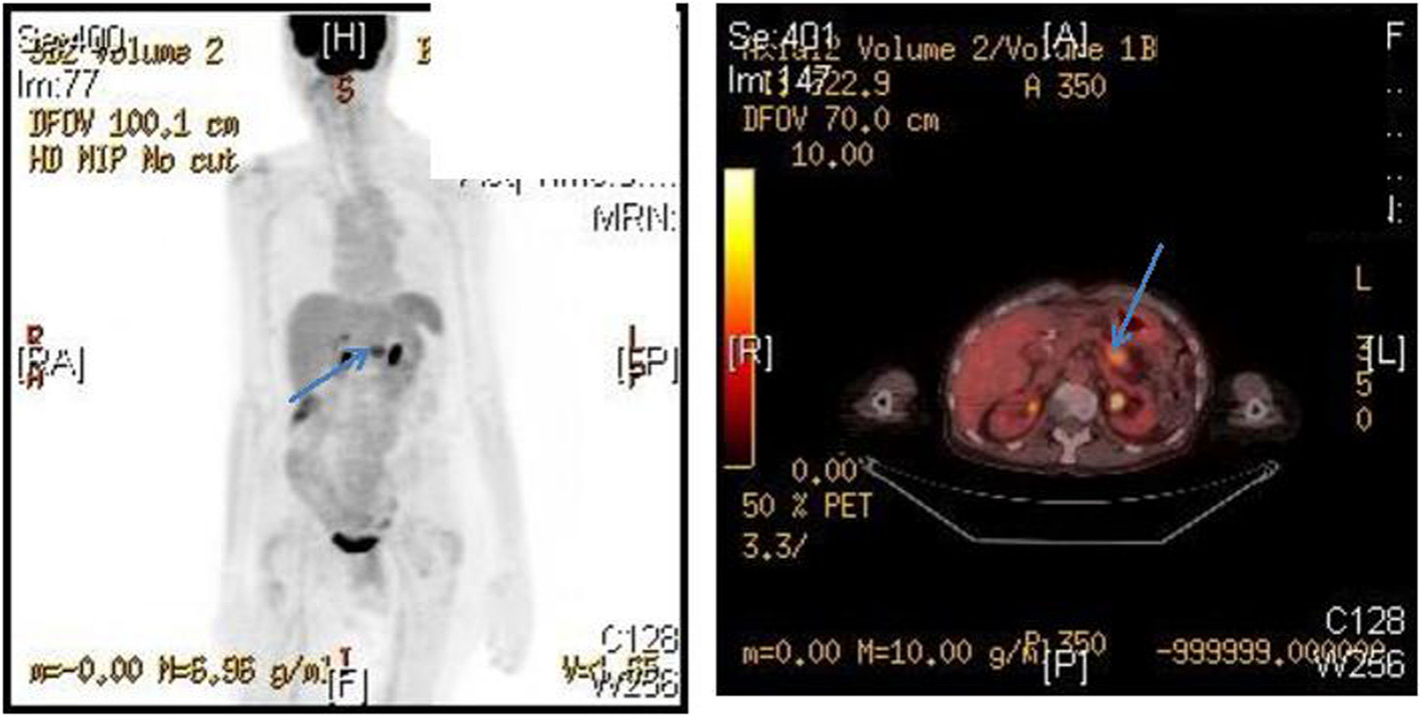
PET (a) and PET-CT (b) images demonstrating the mass within the pancreatic remnant with no evidence of metastatic disease
